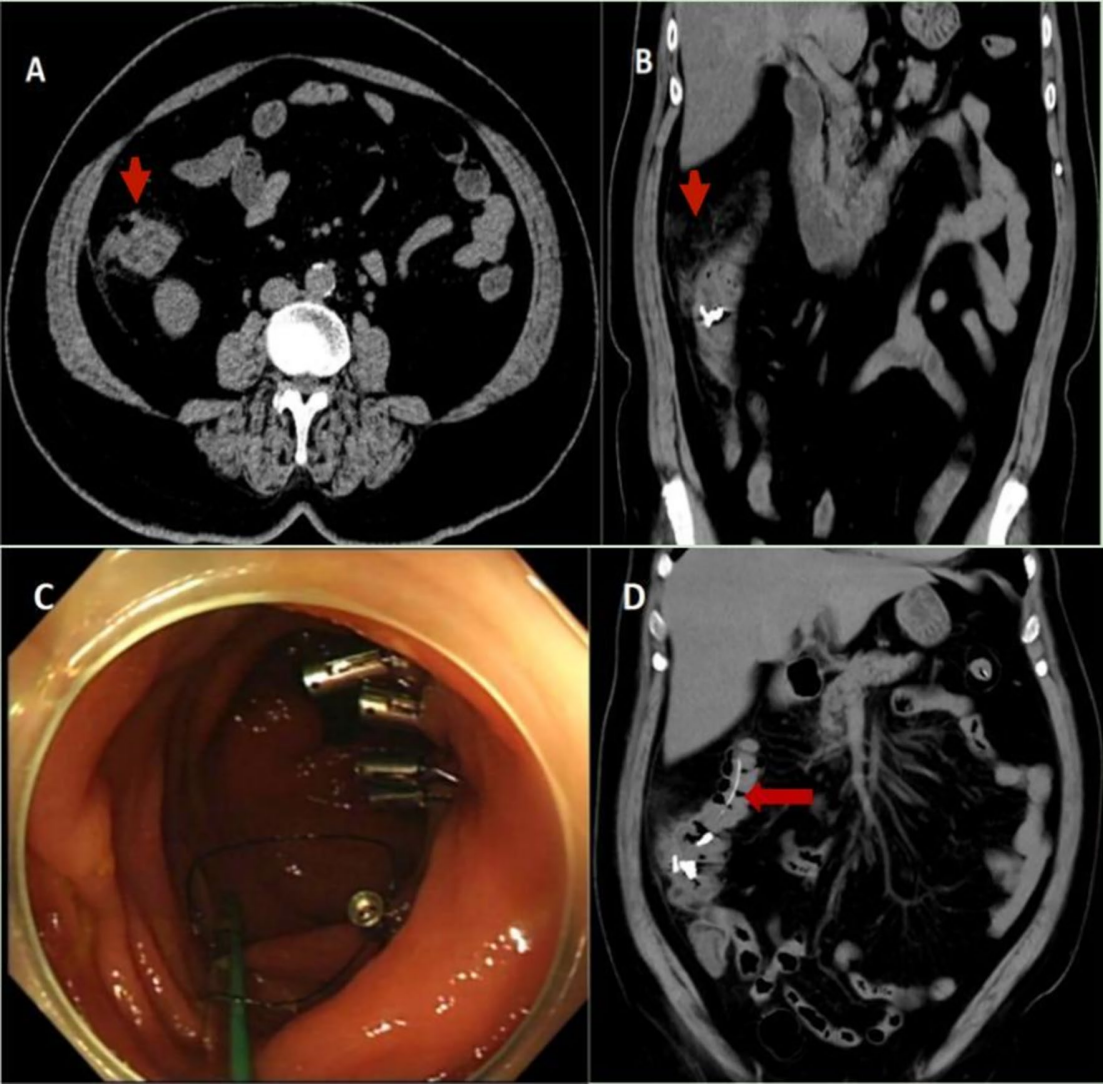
A–B Abdominal CT shows gas and inflammatory exudation around the ascending colon (red arrow). C Two additional endoclips applied to secure the wound, the colonic TET placed and fixed near the wound. D Follow-up CT showed exudation getting better after TET drainage (red arrow: colonic TET)
